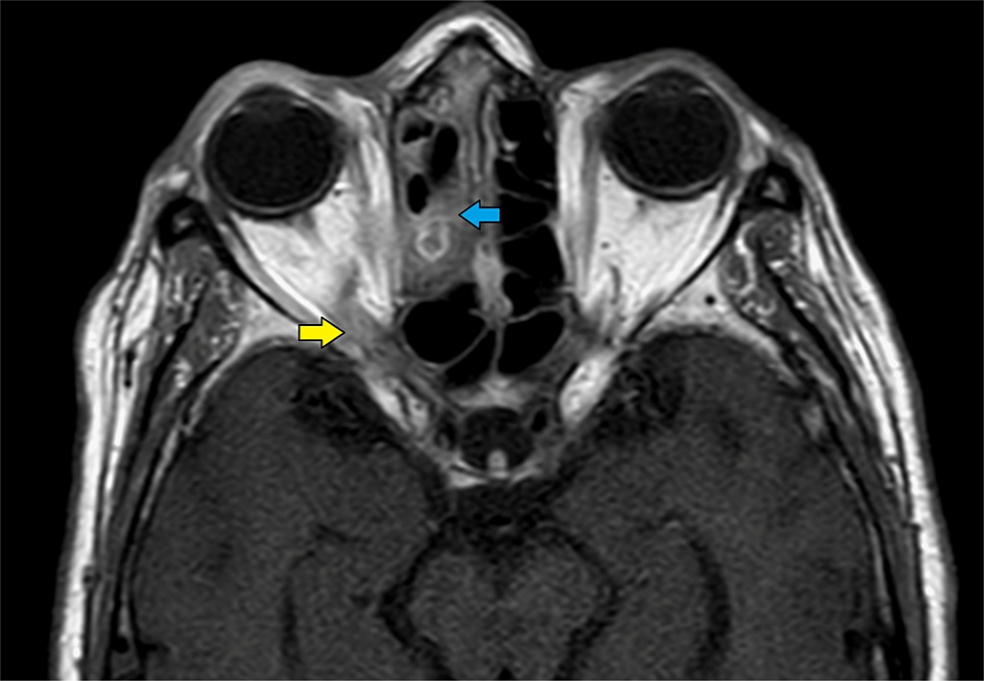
Magnetic resonance imaging (T1 post-contrast axial section) showing heterogeneous signals in the right orbital apex with focal extension into the cavernous sinus (yellow arrow) and heterogeneous enhancement of the mucosa along with fluid in the right ethmoid air cells (blue arrow).
